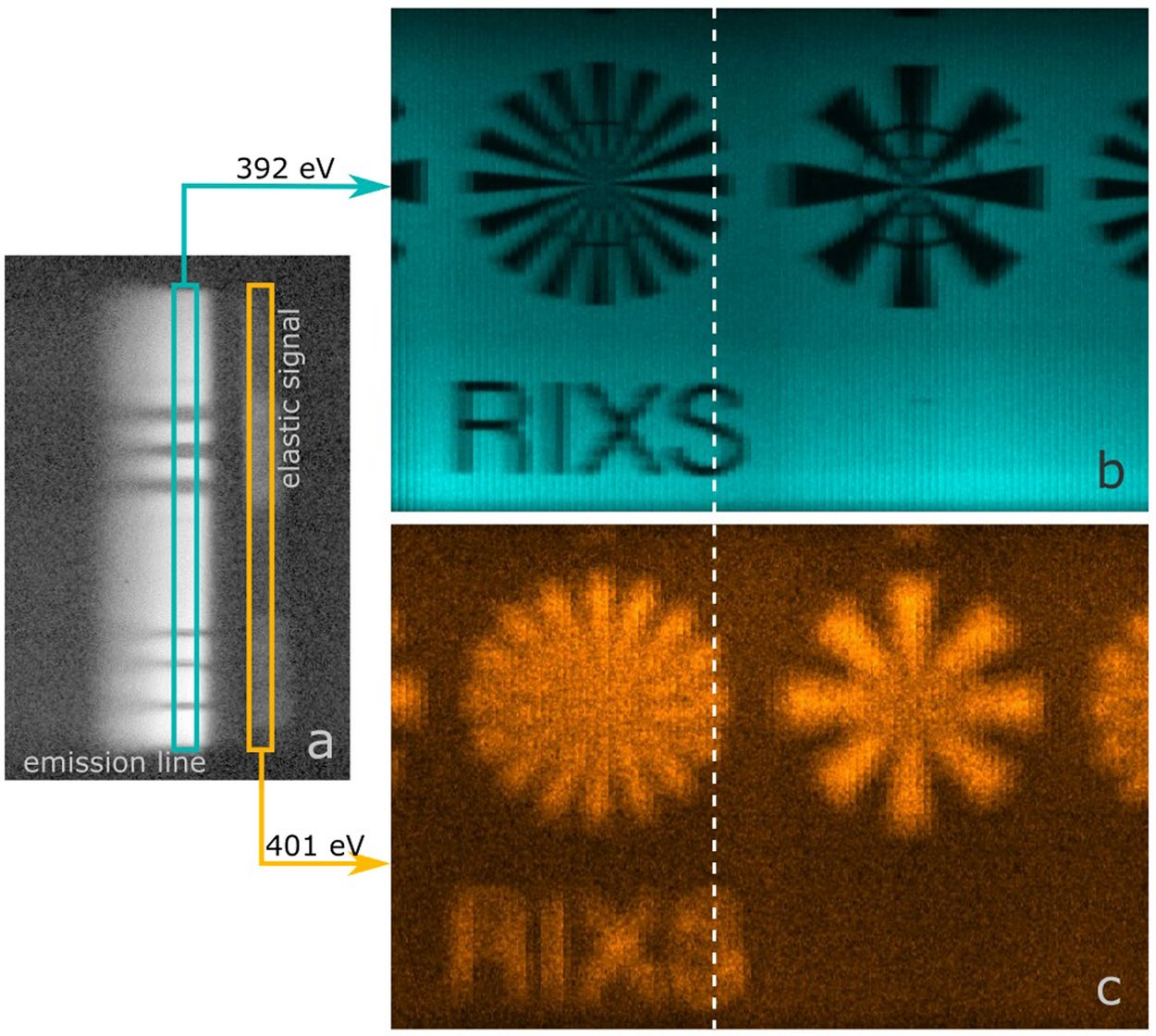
(a) RIXS signal of a test sample illuminated with a line focus of 10 μm(h) × 1000 μm(v) at a mean incident photon energy of 401 eV ± 0.35% (elastic peak of Si_3_N_4_) and an exposure time of 20s. (b) and (c) are reconstructions out of 101 such images, whereby (b) is reconstructed from the inelastic Si_3_N_4_ X-ray emission signal and (c) from the elastic signal. The dashed line in (b) and (c) indicates the position of the signal (a). The sample was a silicon wafer coated with 250 nm Si_3_N_4_ and 20 nm thick Au patterns. The diameter of the Siemens star was 500 μm. In the left star with the finer spokes, the outer ring structure marks structure width of 25 μm whereas the inner ring marks structure width of 10 μm.
